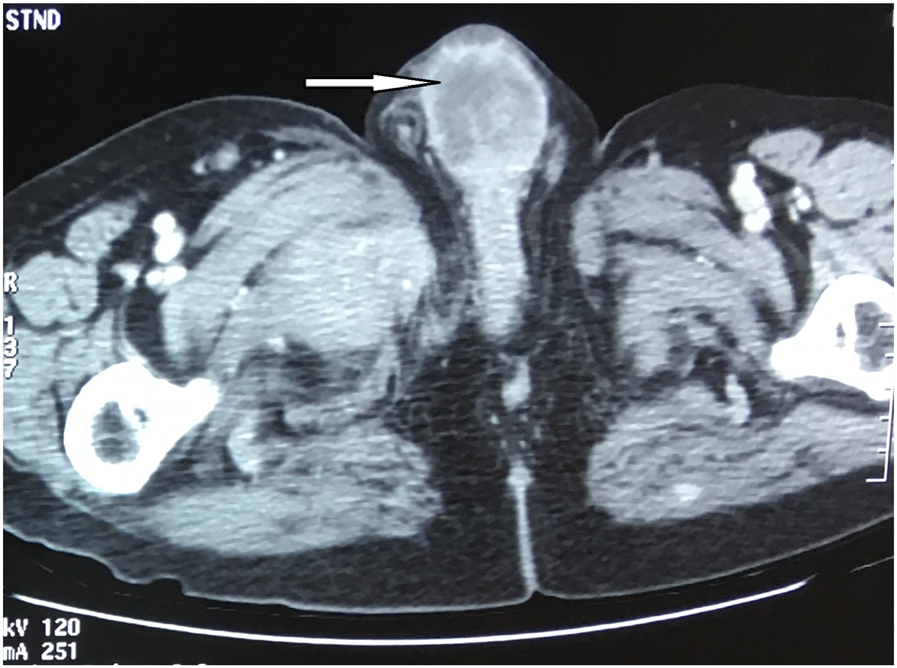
Computed tomography scan showing the penile metastasis (arrow)
